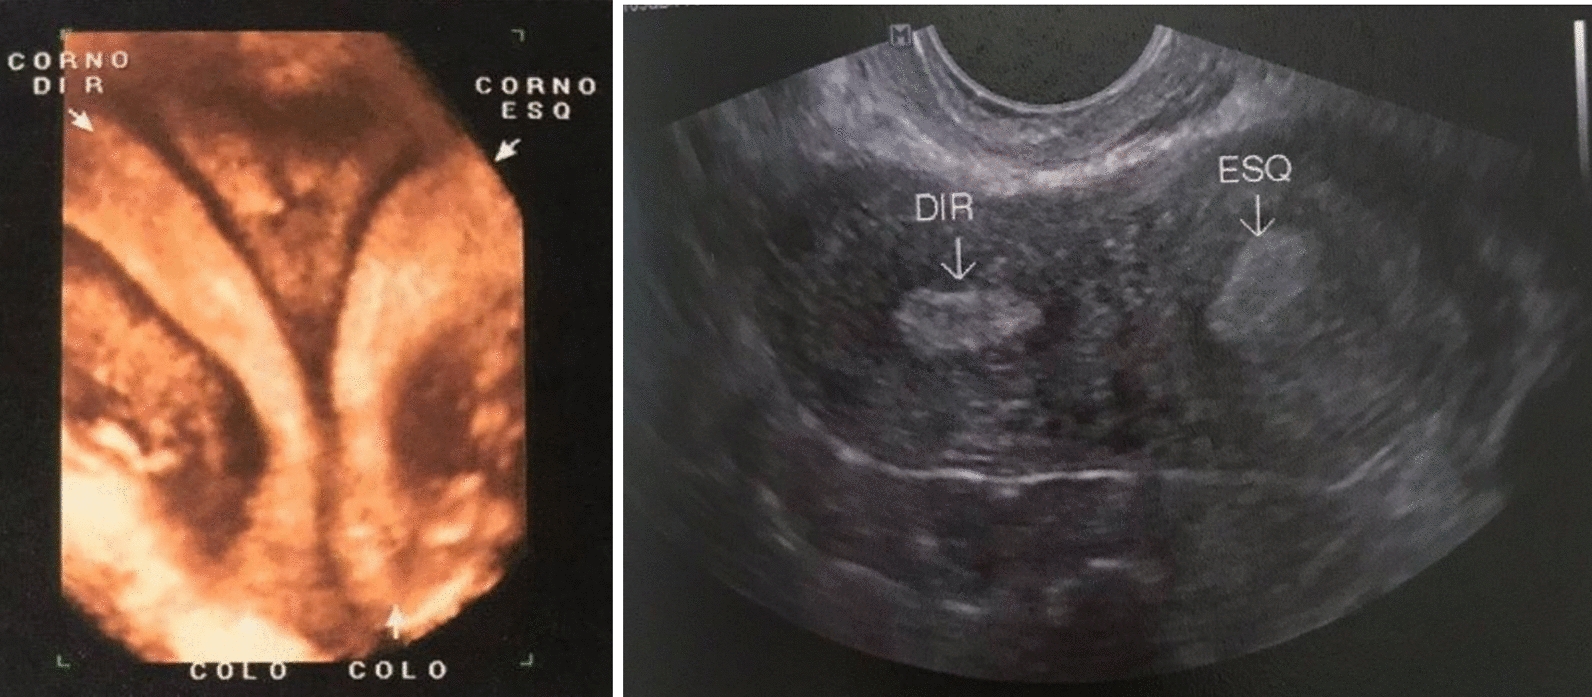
Ultrasound showing the didelphys uterus at diagnosis. In the image on the left, the right (“Corno dir”) and left horns (“Corno esq”) can be visualized, as well as the two cervical canals (“colo”) of the patient, marked in the lower region of the image. On the right, we can see the didelphic uterus, with arrows pointing to the right (“DIR”) and left (“ESQ”) uteri
